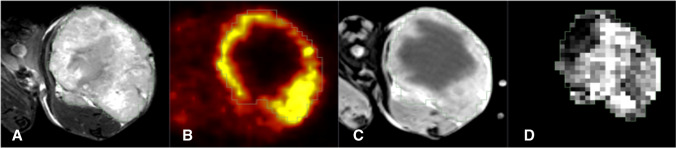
Multi-dimensional pre-clinical imaging data. Example of pre-clinical imaging data consisting of A anatomical T2-weighted MRI, B FMISO PET, and C contrast-enhanced T1-weighted MRI, D apparent diffusion coefficients (ADC) derived from diffusion-weighted (DW) MRI
